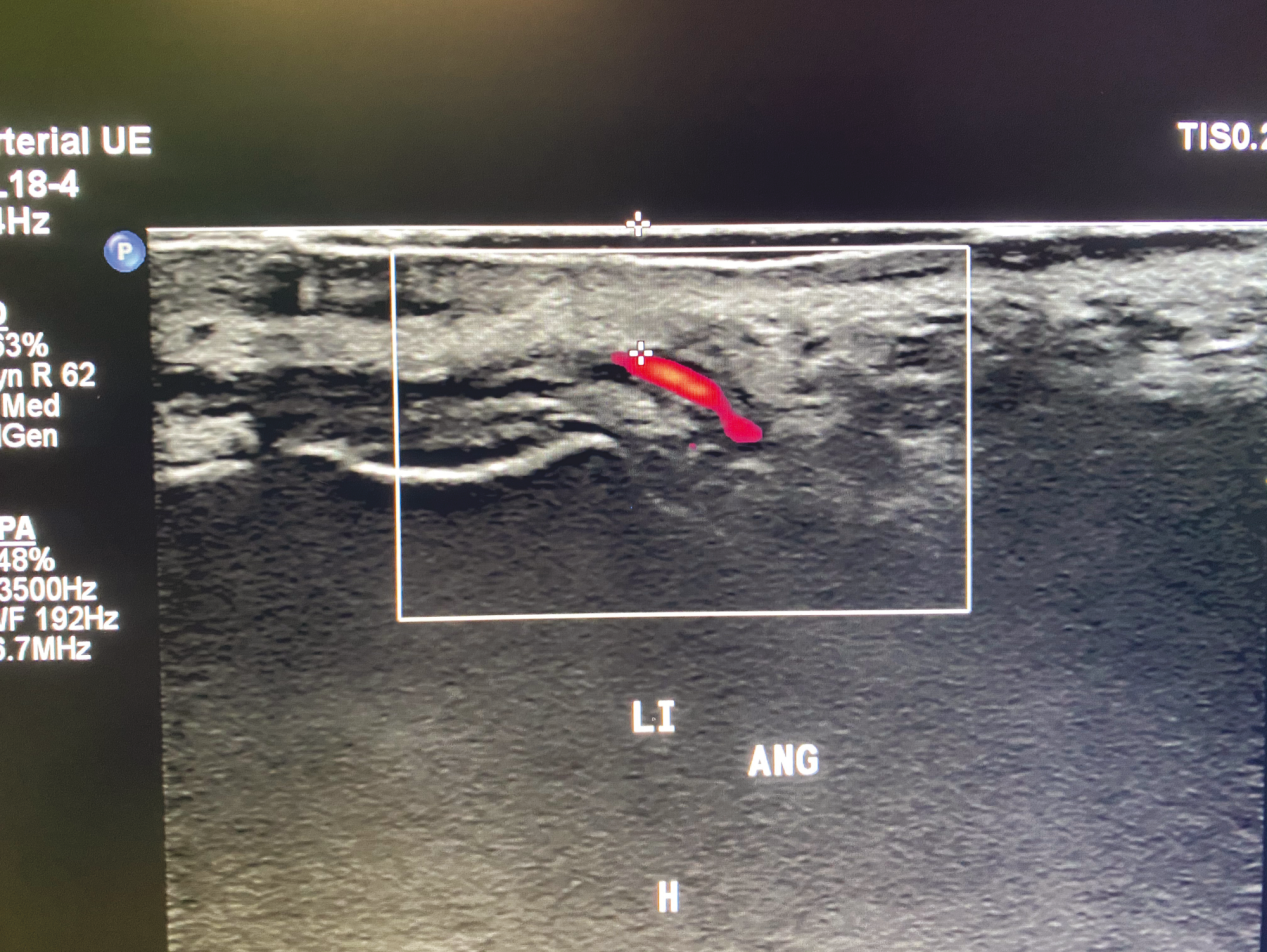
View of the left angular artery using duplex ultrasound. H, head; Li Ang, left angular artery.
